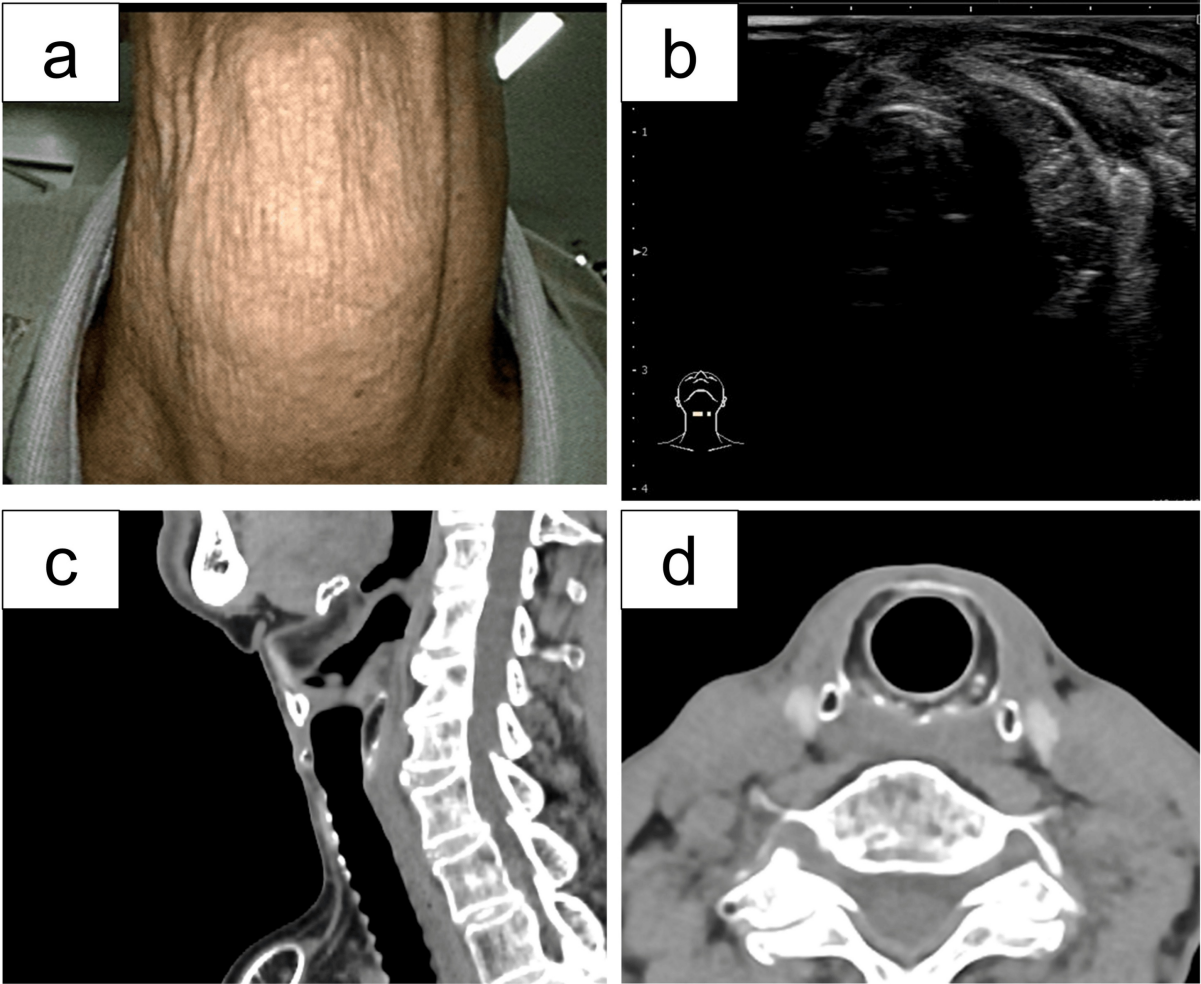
Postoperative clinical course (a) Local findings. Examination of the surgical site revealed no swelling, erythema, tenderness, or other abnormalities. (b) Ultrasonographic findings. Postoperative ultrasound of the anterior neck revealed no evidence of residual lesion or recurrence. (c, d) Computed tomography (CT) findings. Sagittal (c) and axial (d) CT images demonstrated no signs of local recurrence.
